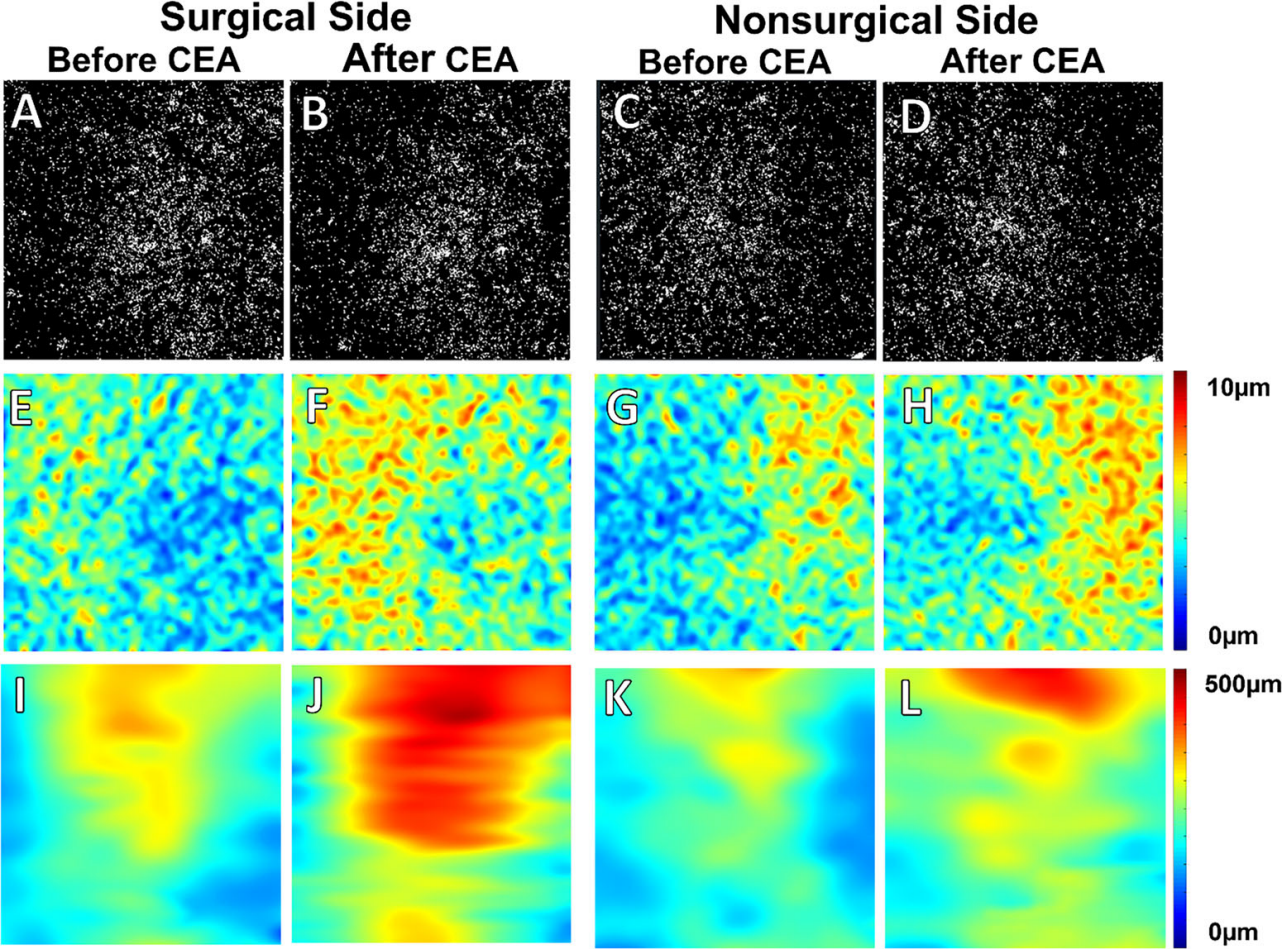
Case 2. A 67-year-old subject with 85% CAS on the surgical side and 50% CAS on the nonsurgical side underwent unilateral CEA on the ipsilateral surgical side. (A–D) CC FD% maps. (E–H) CC thickness maps. (I–L) CT maps. On the surgical side, no visually significant difference was seen between CC FD% maps before and after CEA, although the CC FD% measurement had a minor decrease after CEA: 12.09% (A) versus 11.35% (B). After the CEA, there was a significant increase in the CC thickness (8.08 µm before CEA vs. 10.47 µm after CEA; E vs. F) and in the CT (250.17 µm before CEA vs. 366.11 µm after CEA; I vs. J) maps. On the nonsurgical side, there was no observable change in the CC FD% maps before and after CEA (11.15% before CEA vs. 10.92% after CEA; C vs. D). The CC thickness map showed a less prominent but noticeable increase after CEA (8.62 µm before CEA vs. 9.84 µm after CEA; G vs. H), with a similarly observable increase in the CT map superiorly (227.11 µm before CEA vs. 296.22 µm after CEA; K vs. L).
